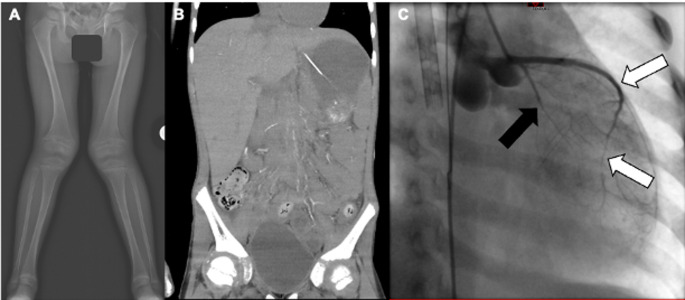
(A) Radiographs of a child with ENPP1 deficiency at age 4 years 11 months demonstrated genu valgus deformity and rachitic changes at the growth plate of the distal femur, proximal and distal tibia and fibula. (B) Abdominal CT of the same child at age 6 years 5 months demonstrating mesenteric artery calcifications. (C) Selective left coronary artery angiogram showing diffuse distal left circumflex (black arrow) and left anterior descending hypoplasia (white arrows) and irregularity with near-obliteration of flow
